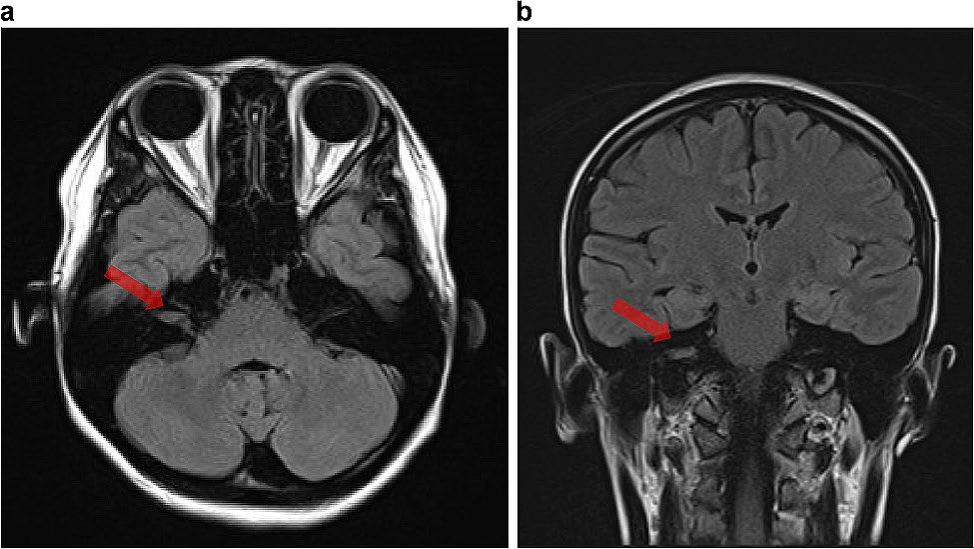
A mass (arrow) in the right auditory canal on axial (a) and coronal (b) FLAIR.
